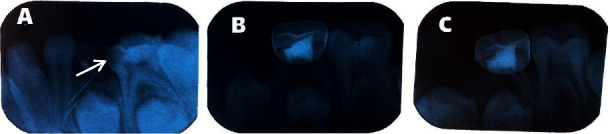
(a) Pretreatment radiography, (b) external root resorption in 6-months follow-up, and (c) external root resorption in 12-months follow-up (treated tooth is marked with an arrow).
